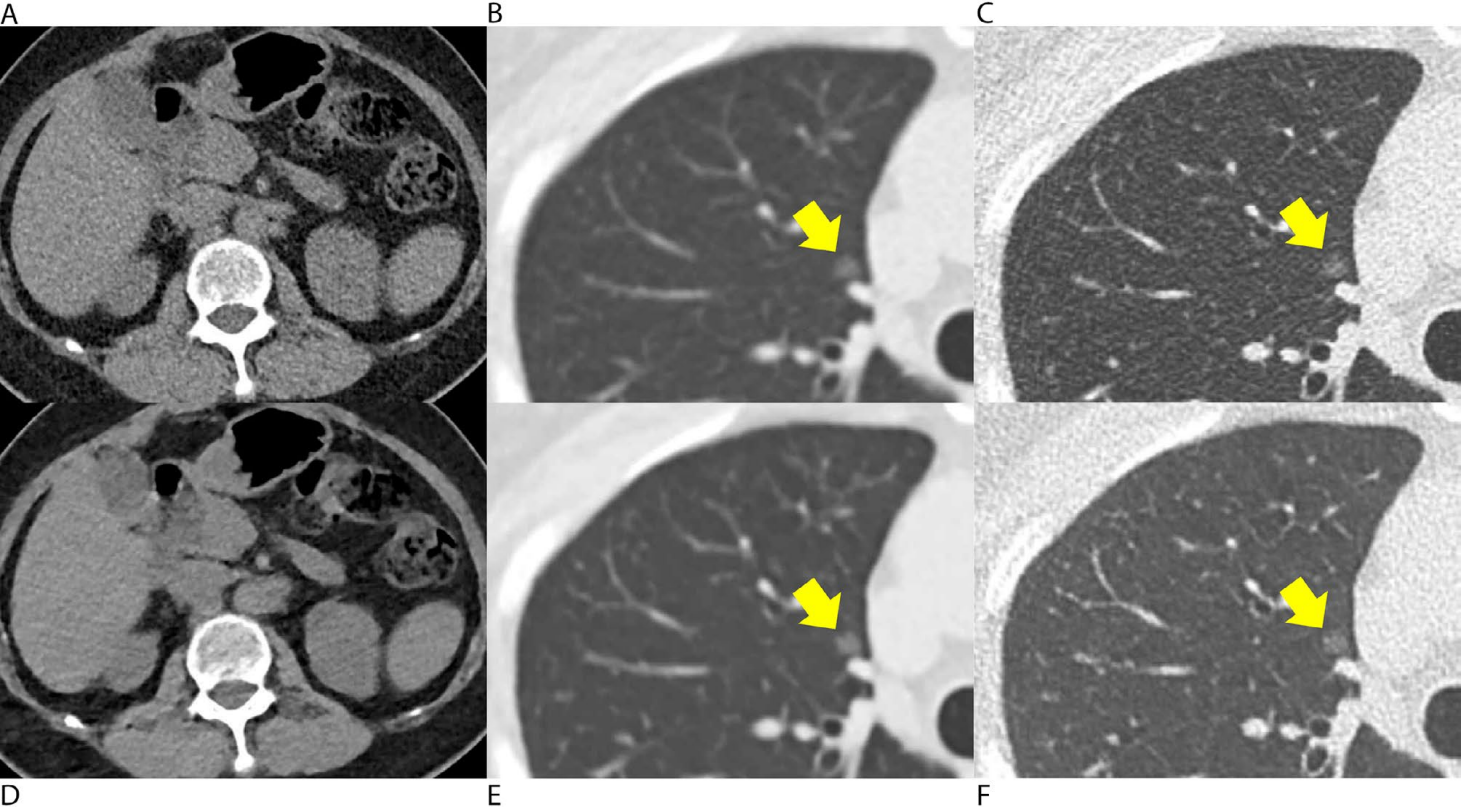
A woman received low-dose chest CT for the follow-up evaluation of multiple ground-glass nodules. (A-C) Conventional low-dose chest CT images were reconstructed with iterative reconstruction, and (D-F) the images generated using a quarter dose of radiation were reconstructed with commercial deep-learning software. (A, D) Soft-tissue structures, including the liver, spleen, kidney, and paraspinal muscles, were visualized with a lower noise level in the mediastinal-window setting on both images. (C, F) A ground-glass nodule in the right upper lobe was well visualized with a sharp margin on both images (arrow)
